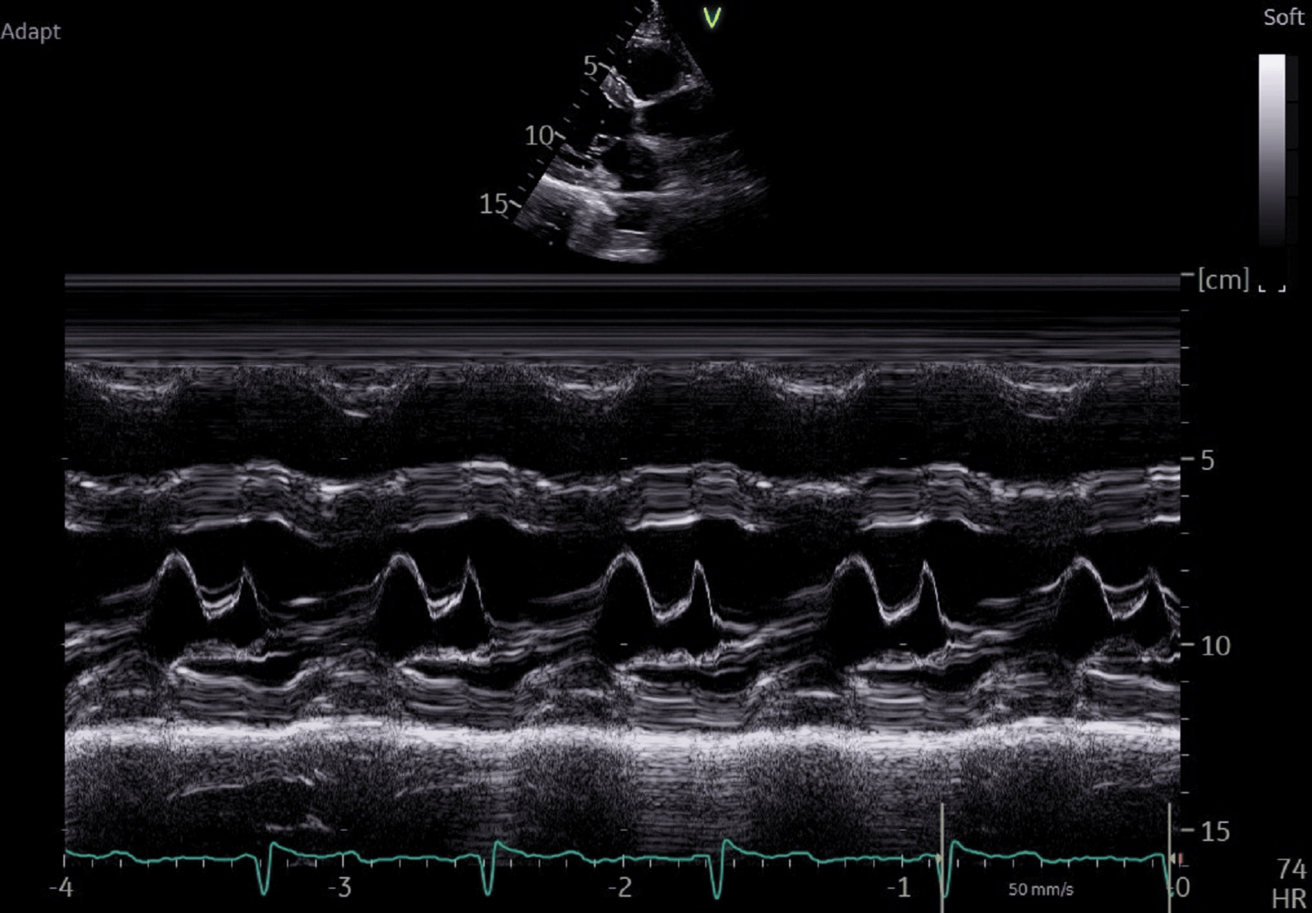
TTE showing an absence of SAM of the mitral valve, which means an absence of LVOT obstruction TTE: trans-thoracic echocardiography, SAM: systolic anterior motion, LVOT: left ventricle outflow tract
